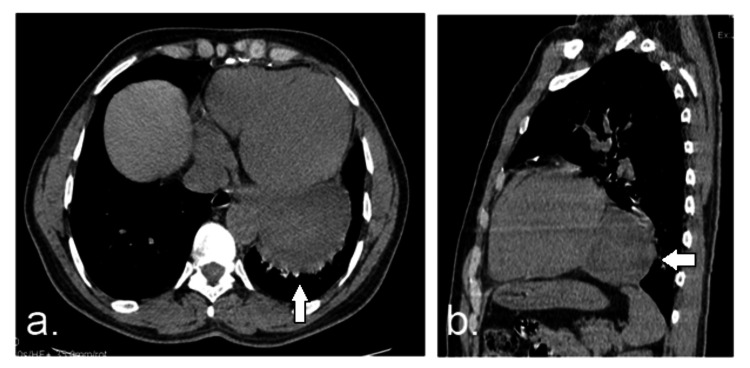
(a) axial and (b) sagittal unenhanced CT (mediastinal window) images show the fluid density lesion located in the left posterior cardiopheric angle in close proximity to the pericardium (white arrow)
